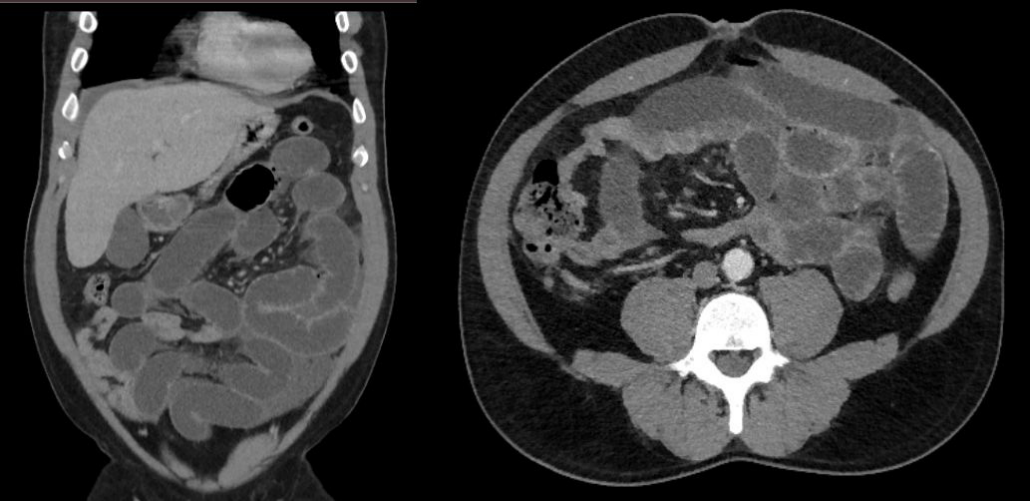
Abdominal Computed Tomography (CT) scan, which showed distended bowel segments and signs suggestive of edema of small bowel loops (thickening of the small bowel wall).
